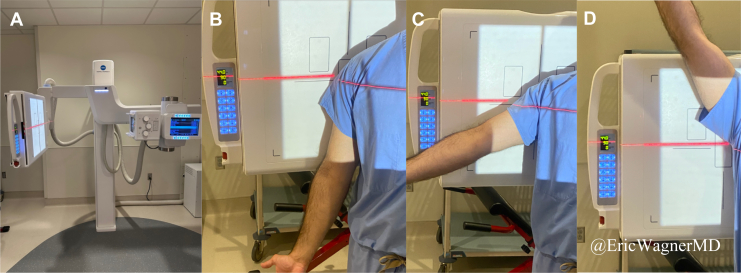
Konica Minolta dynamic digital radiography (DDR) machine (A). Patients are positioned in a Grashey view with their posterior shoulder and scapula contacting the back board of the DDR machine. Patients are instructed to stand upright, stationary, avoid axial rotation, and how to perform the motion including standardizing for forearm supination. Patients begin with their arm at rest and gradually abduct their arm to maximum elevation (B-D).
